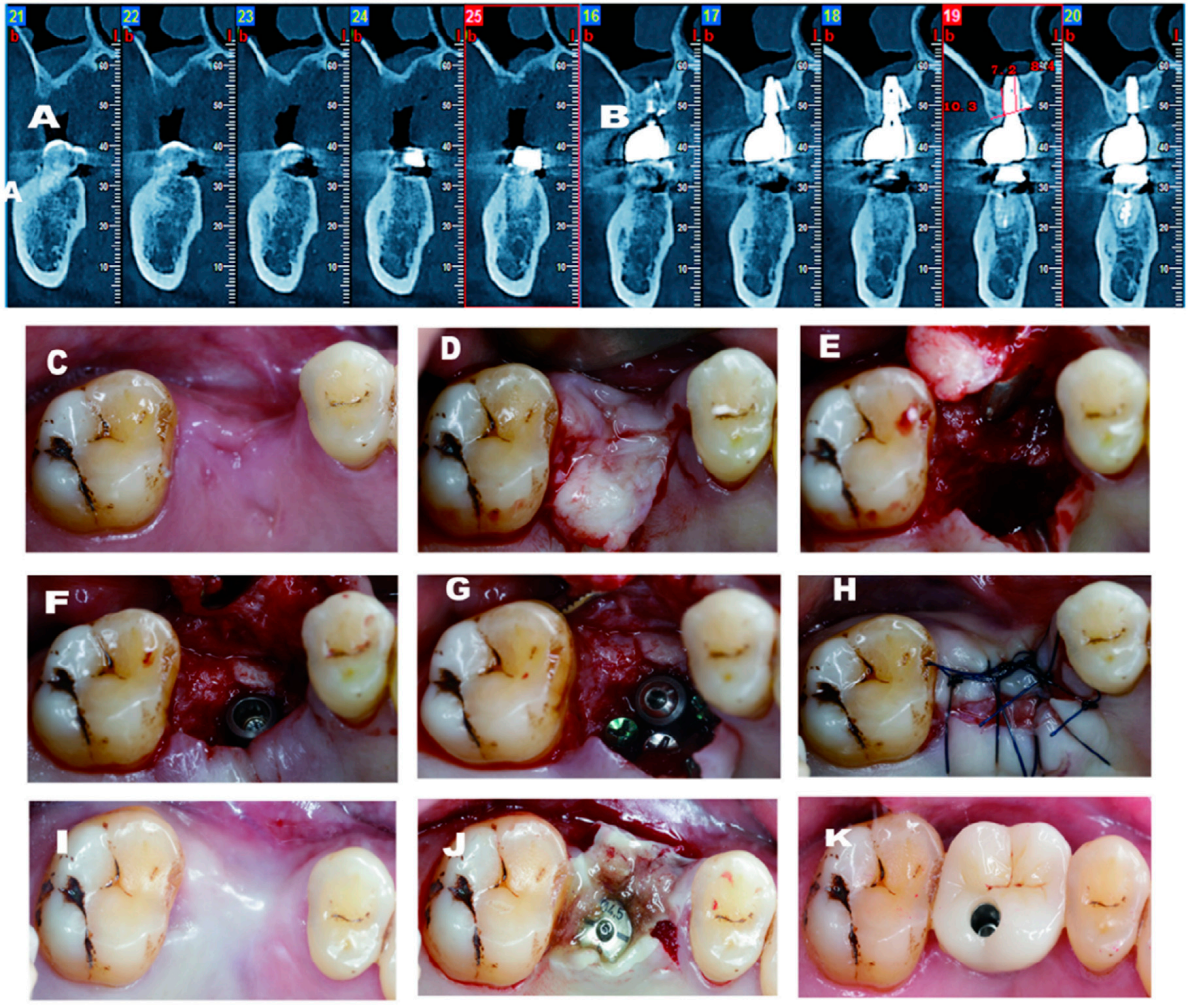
(A) Pre-operative CBCT demostrates large palatal and vertical defect; (B) 3 years follow-up CBCT shows excellent gain on both vertical and palatal aspects; (C) Pre-operative-occlusal view; (D) Palatal flap elevated; (E) Significant palatal defect after chipped palatal bone; (F) Implant installed after sinus floor transportation; (H) Non-primary closure after GBR; (1) 4 months after surgery; (J) Second stage surgery with tissue glew; (K) Final crown deliv- ery.
